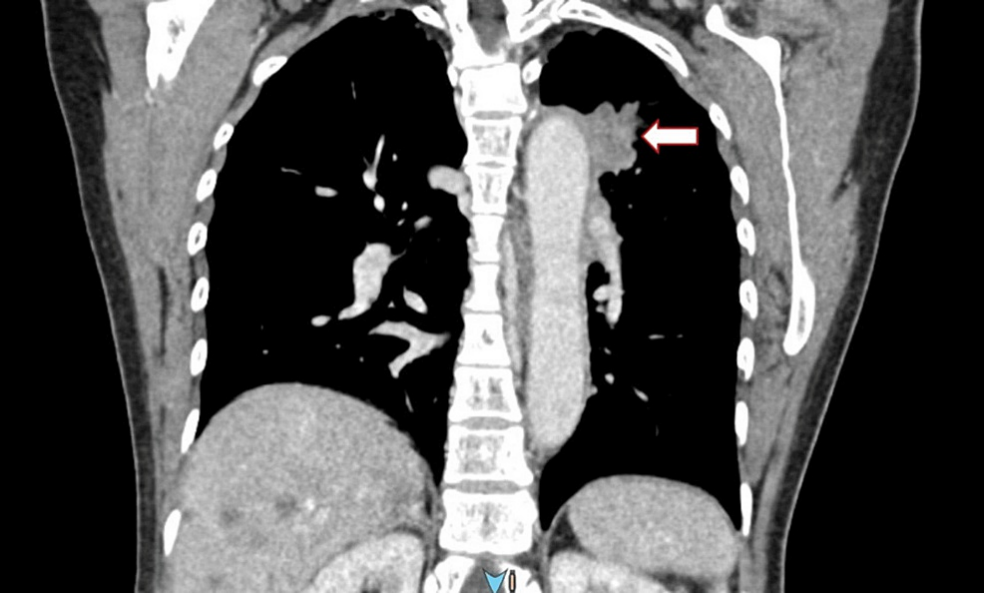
CT thorax showing irregularly shaped mass in the apicoposterior segment apical of the left upper lobe measuring 4.6 x 3.0x 1.9 cm (length x width x AP)
